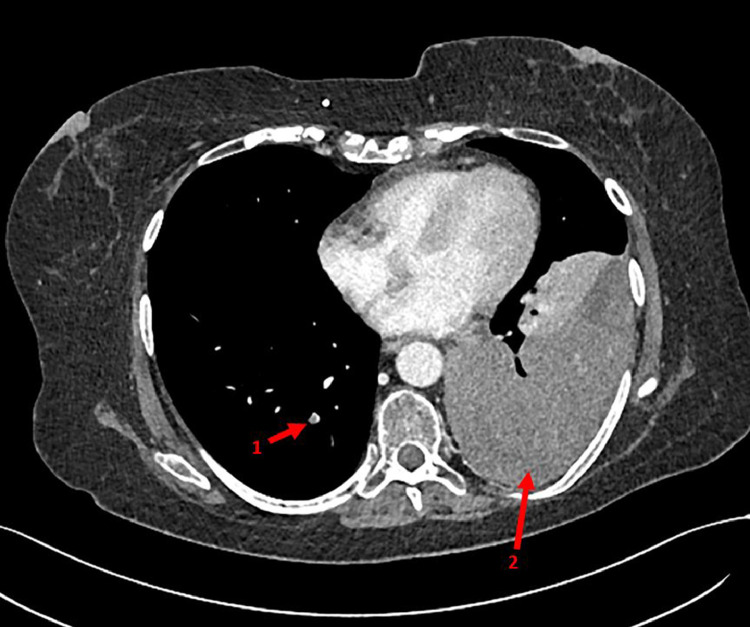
Chest computed tomography pulmonary angiography at presentation; central and right-sided subsegmental pulmonary embolisms (arrow 1), and a pleural tumour in the left lower lobe (arrow 2).
